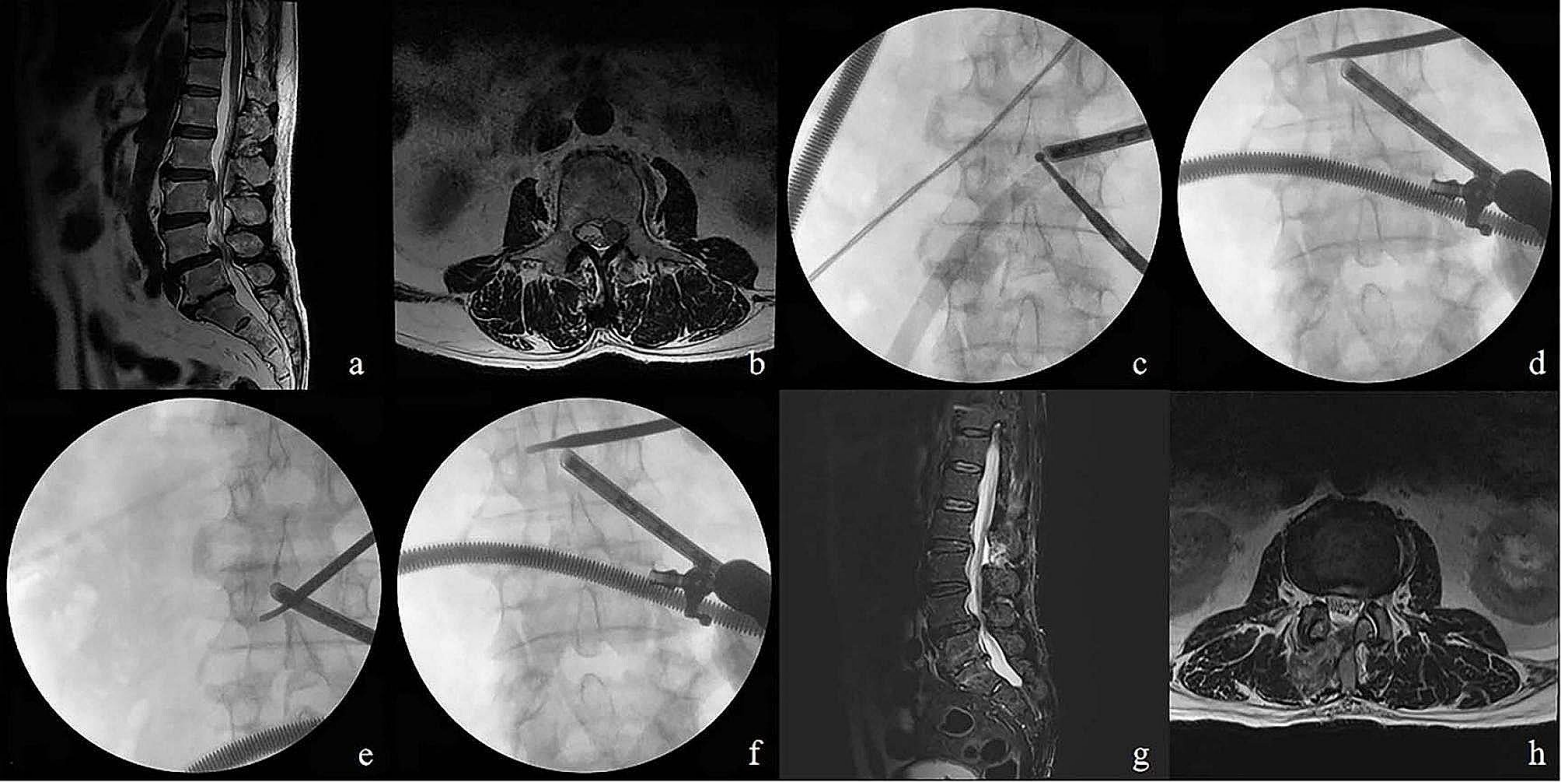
L2/3 down migrated disc shown in preoperative T2-weighted sagittal magnetic resonance imaging in sagittal (a) and axial views (b). Desired initial tagert portal position in X-rays (c). X-ray confirms that the tool is operated to the opposite side (d). Intraoperative fluoroscopicimage confirming inferior (e) and superior (f) of pedicle and complete decompression of traversing nerve root. Complete removal of ruptured disc without remnant disc material in postoperative MR images in sagittal (g) and axial views (h)
